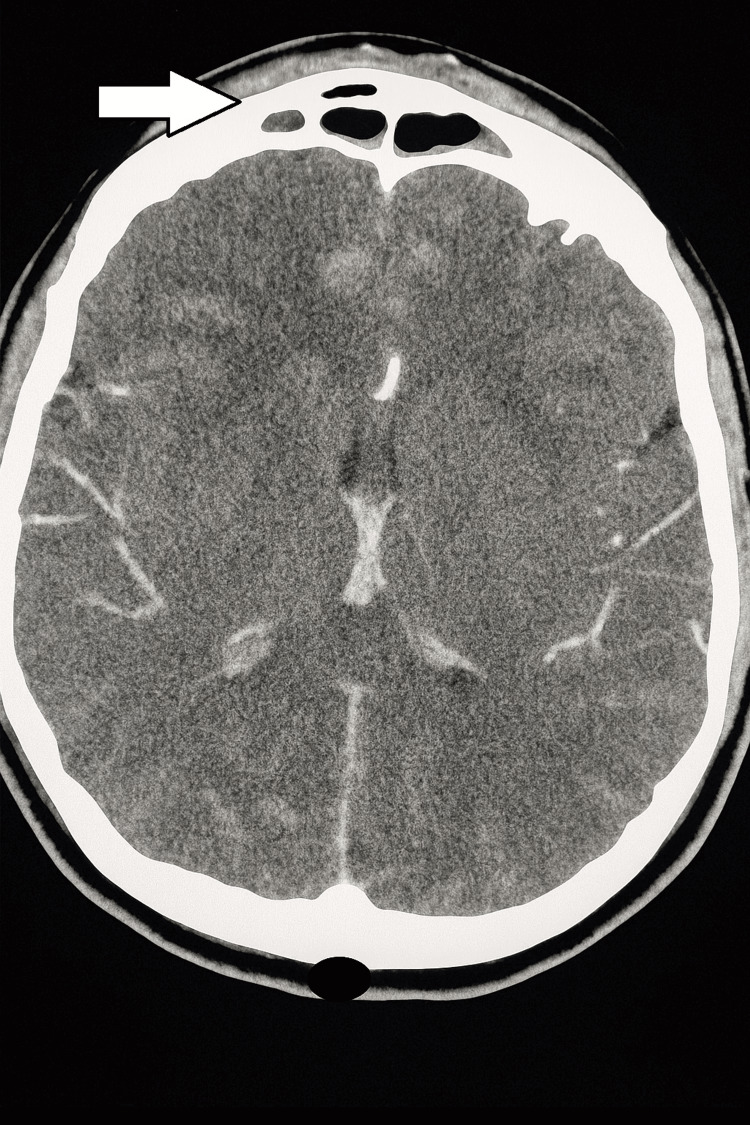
Head CT with contrast showing bony erosion of the frontal sinus with fluid collection within the sinus cavity (white arrow) and no intracranial extension.
